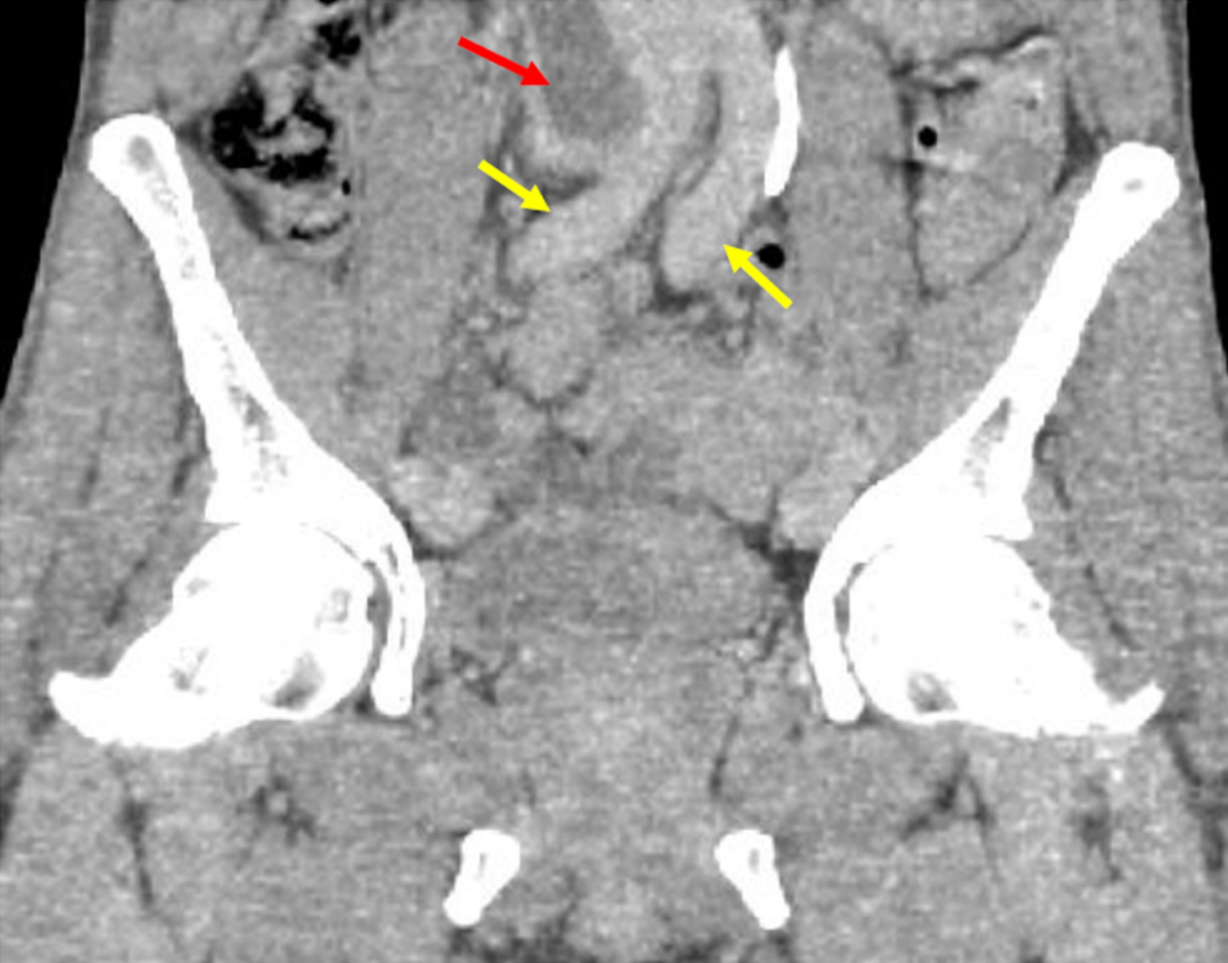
Coronal Computed Tomography Demonstrating Filling Defects within the Confluence of the Inferior Vena Cava Coronal computed tomography images of the abdomen and pelvis demonstrate the bilateral common iliac arteries (yellow arrows) and filling defect (red arrow) within the confluence of the inferior vena cava, consistent with a large thrombus.
